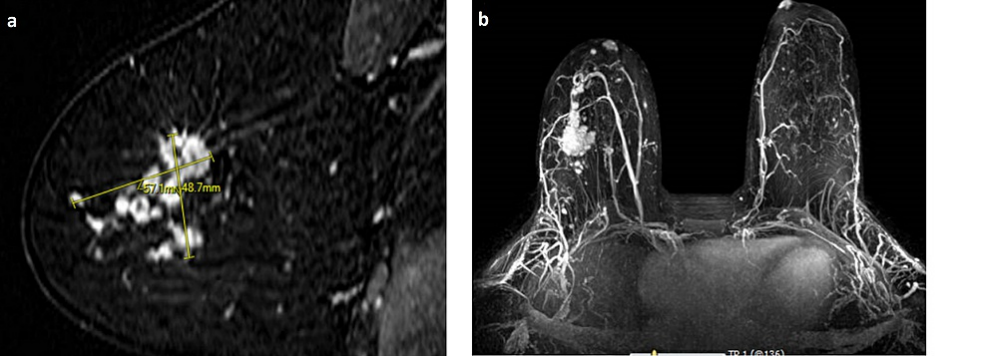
(a) Magnetic resonance imaging of the right breast with heterogeneous mass with irregular margins measuring 57.1 mm x 48.7 mm. (b) Magnetic resonance imagining with contrast in axial view.
